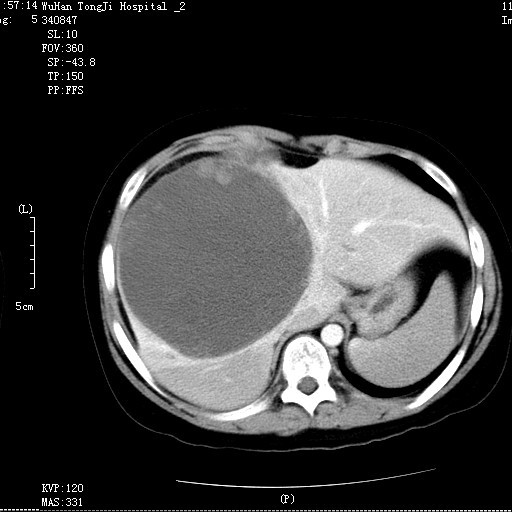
Contrast enhanced CT scan showing hepatomegaly and a large hypodense lesion in the liver.
